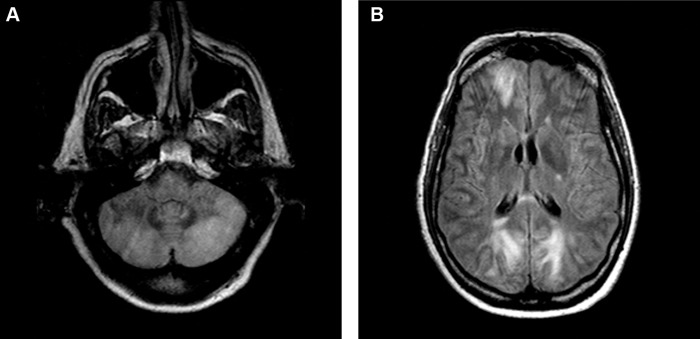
Repeat MR imaging (FLAIR sequence) demonstrating more extensive involvement of the cerebellum (A) and oedema in the subcortex of the frontal and occipital lobes (B) consistent with PRES.
